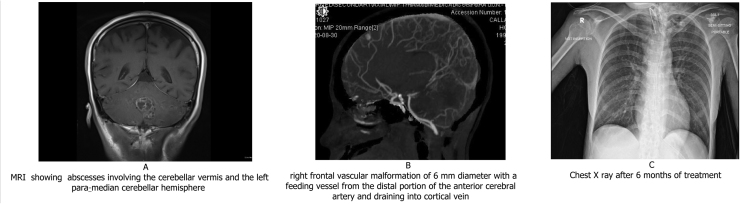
(A) MRI showing abscesses involving the cerebellar vermis and the left para-median cerebellar hemisphere (B) right frontal vascular malformation of 6 mm diameter with a feeding vessel from the distal portion of the anterior cerebral artery and draining into cortical vein (C) Chest X ray after 6 months of treatment.
